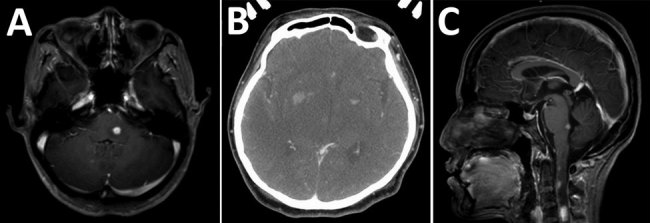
Imaging of brain and spine of 3 patients infected with *Mycobacterium haemophilum* who had involvement of the central nervous system, Bangkok, Thailand. A) Patient 1, axial T1-weighted magnetic resonance imaging scan with gadolinium showing enhanced nodule at left dorsal pons. B) Patient 2, axial contrast-enhanced computed tomography scan showing hypodensity lesions in both thalami and nodular enhancement at the bilateral basal ganglia. C) Patient 3, sagittal T1-weighted magnetic resonance imaging scan with gadolinium showing multiple enhancing nodules at dorsal pons and upper cervical cord.
